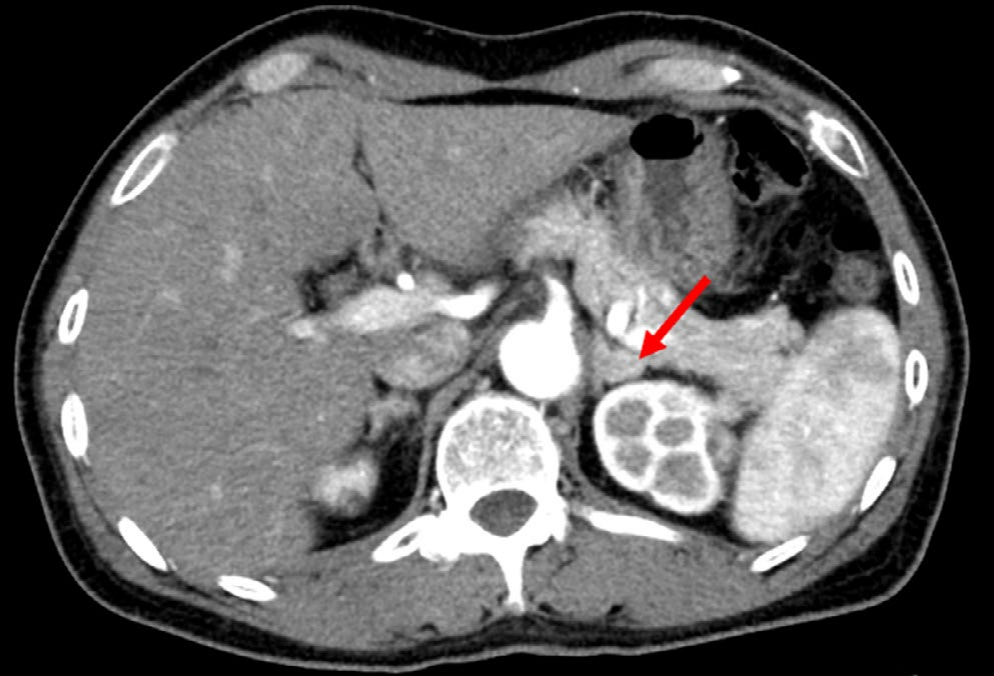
Abdominal computed tomography scan showed an approximate 13 mm left adrenal mass (red arrow)
